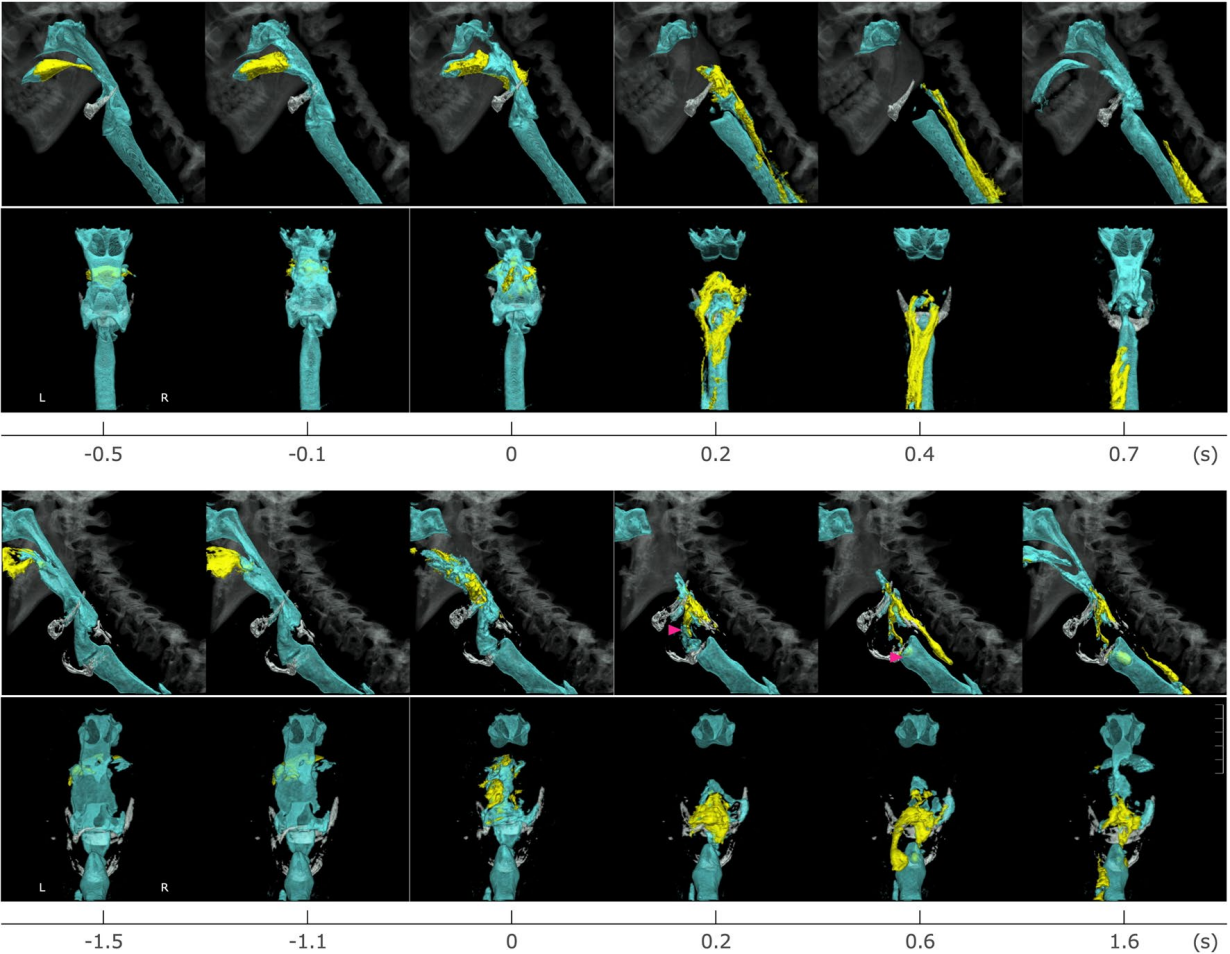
3D-CT images of swallowing in healthy volunteer (upper two rows) and in dysphagia patient (lower two rows). In both swallows, upper row is lateral view and lower row is posterior view. Yellow is bolus and blue is air column surface. Arrows show the penetration and aspiration
